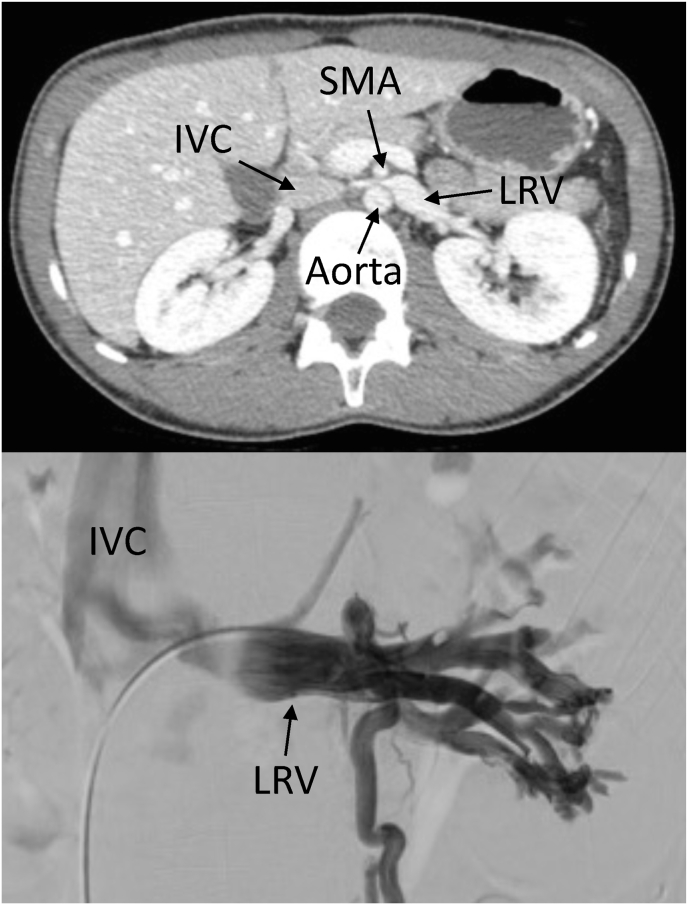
Contrast-enhanced computed tomography (CT) and renal venography showing entrapment of the left renal vein (LRV) between the aorta and superior mesenteric artery (SMA) prior to its insertion to the inferior vena cava (IVC).
